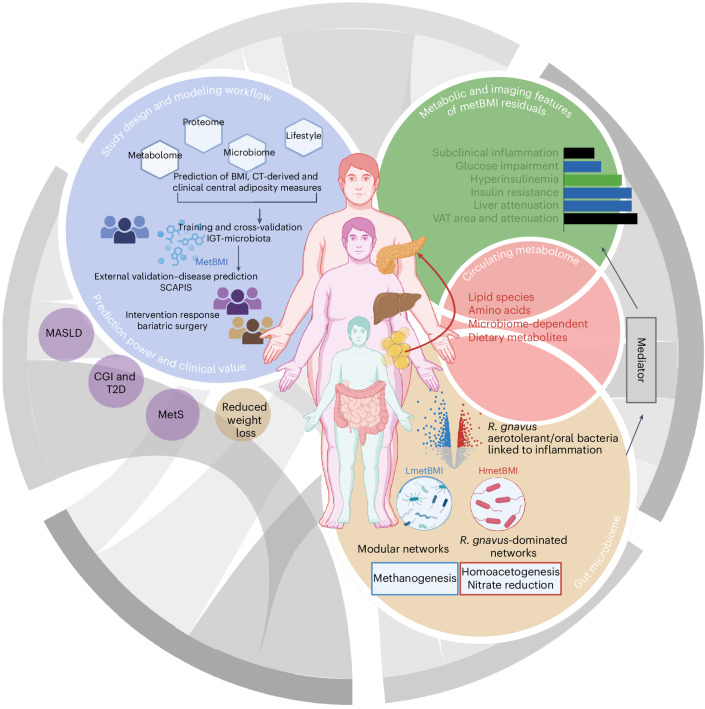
Systems view of metabolic obesity: integrating multi-organ and multi-omics signatures. Light blue circle: deep phenotyping in the IGT-microbiota cohort (*n* = 1,408), including metabolomics, proteomics, metagenomics, diet and clinical profiling, enabled development of metBMI using ridge regression. MetBMI outperformed other omics-based and multi-omics models in capturing central adiposity, explaining over 50% of BMI variance in an external cohort (*n* = 466). In a surgical cohort (*n* = 75), higher metBMI residuals, adjusted for age, sex and BMI, were associated with approximately 30% less weight loss after 1 year. Light green circle: metBMI residuals identified individuals with metabolically adverse obesity, marked by greater VAT area and more severe attenuation, and mediated the relationship between adipose tissue characteristics and insulin hypersecretion. Light taupe circle: these residuals were linked to reduced gut microbial gene richness, altered ecological networks and enrichment of *R. gnavus* and aerotolerant/oral bacteria. Functional shifts included increased nitrate respiration and homoacetogenesis, alongside a reduction in methanogenesis. Light red circle: recursive feature selection and bidirectional mediation analyses identified 116 microbiome → phenotype and 186 phenotype → microbiome paths, primarily mediated by 66 circulating metabolites. This reveals a bidirectional, metabolite-centered interface between the gut microbiome and host metabolism, providing insights into the heterogeneity of obesity and its clinical manifestations. Figure created with BioRender.com. CT, computed tomography.
